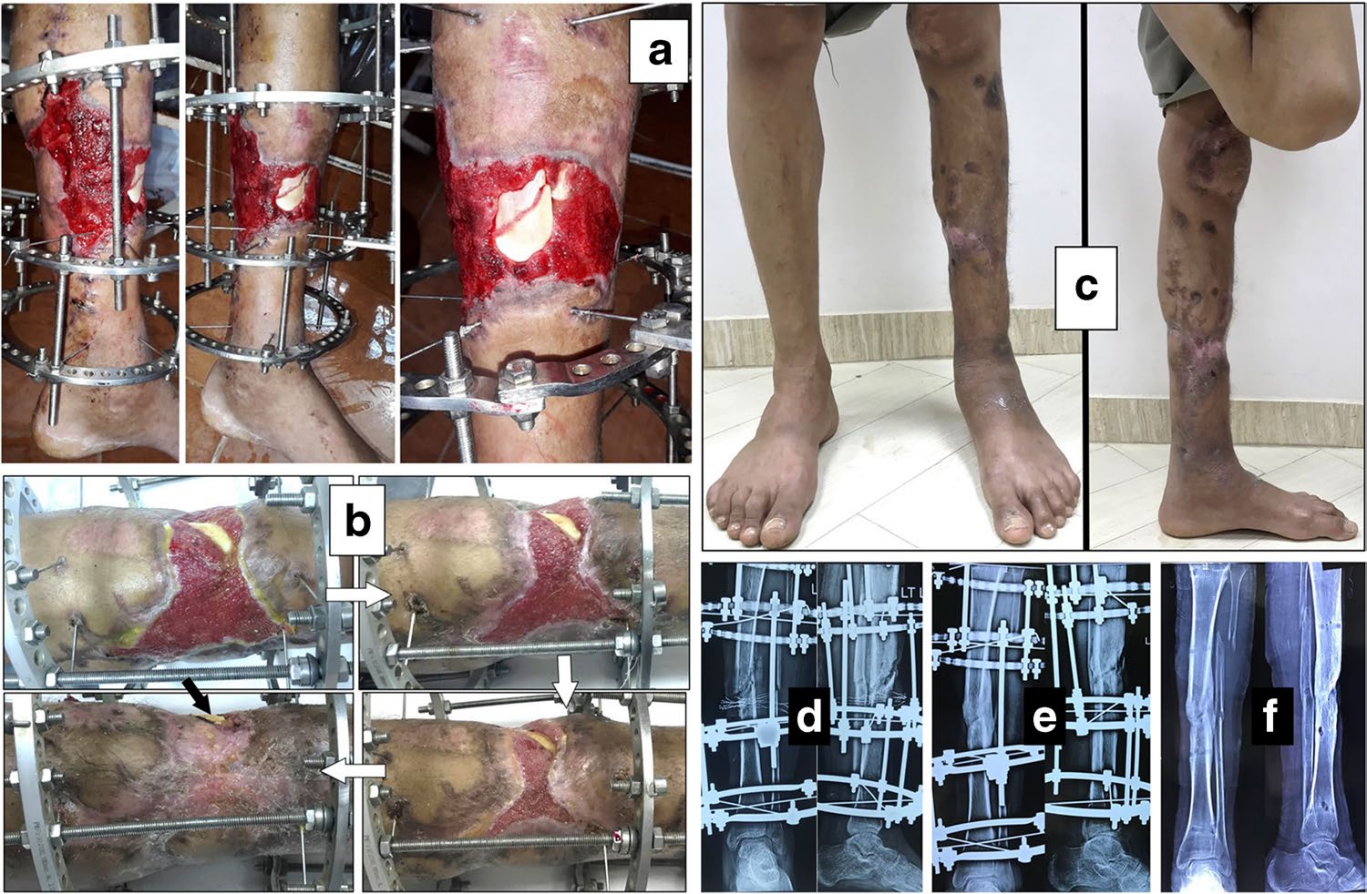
An 18-year-old smoker male patient presented after previous negative pressure wound therapy that was used to treat failed rotational flap in grade III-B open tibial fracture fixed by Ilizarov external fixator. a Different views of wound at presentation with bone exposure. b Clinical photos showing progression of wound repair following the white arrow. The black arrow points to a small anterior sequestered bone flake. c Clinical photos showing the standing patient with dry scar and complete bone coverage. d, e, f Radiographs at presentation, before Ilizarov frame removal with an anterior small sequestrum in the lateral view, and after fixator removal with sound bone union
